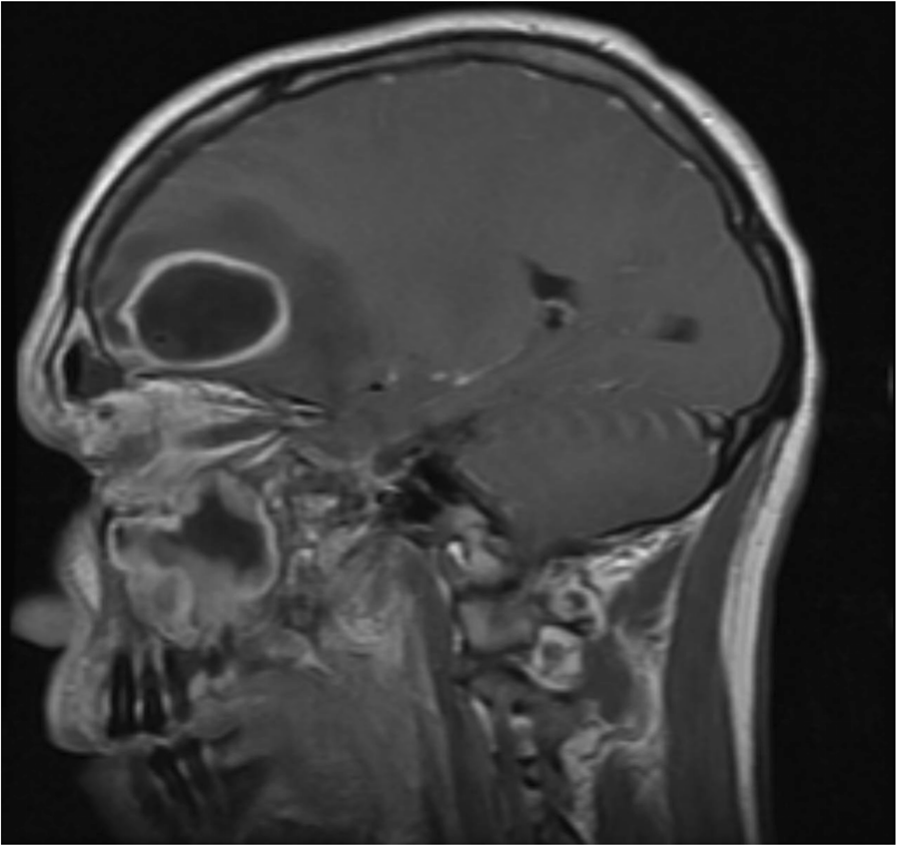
MRI brain, saggital view. Note the hyperintensity extending from the frontal sinus to the abscess cavity
